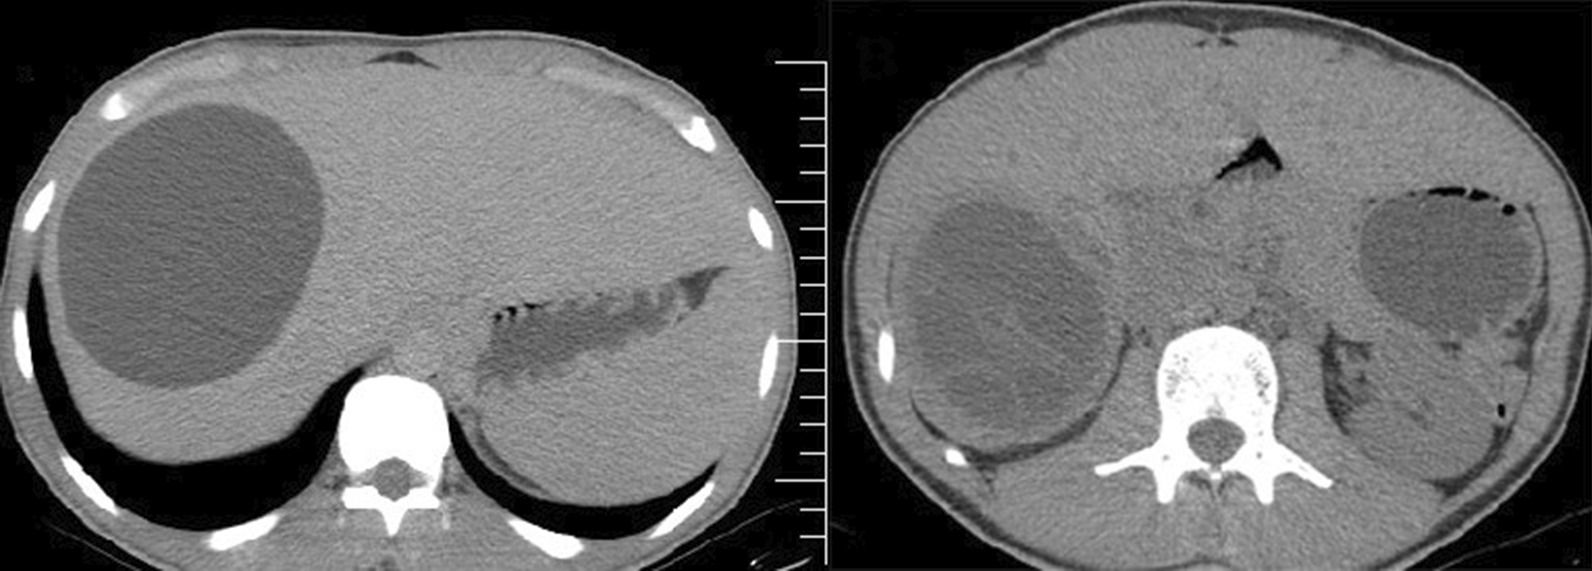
Computed tomography scan of the liver showing two cysts in segments II and VII, respectively, with septation, of which one was intact and the other had ruptured
